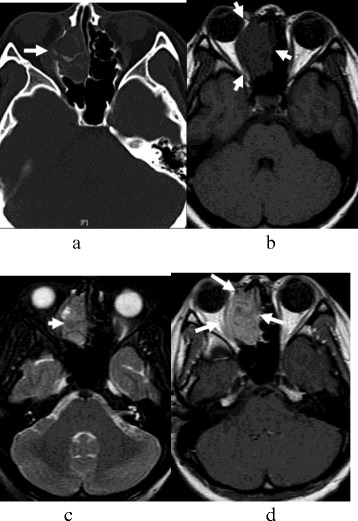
SNEC of paranasal sinuses in a 53-year-old man (a-d). The tumor size was about 4.3 cm × 4.1 cm × 3.1 cm. (a) CT image showed worm-eaten bone destruction in the right ethmoidal sinus and fossa orbitalis; however, bone contours still could be seen. (b) T_1_-weighted MR image demonstrated isointensity. (c) T_2_-weighted MR image demonstrated isointense mixture. (d) Contrast-enhanced T_1_-weighted MR image demonstrated a mild heterogeneous enhancement mass, which showed involvement of the pharyngonasal cavity and fossa orbitalis.
